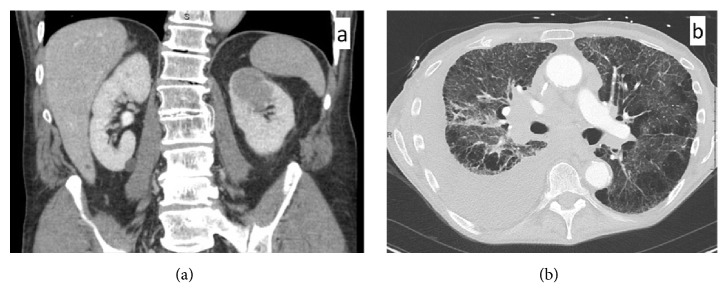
(a) Contrast enhanced CT shows a 4.5 cm left upper pole renal mass. (b) Chest CT shows an ill-defined obstructive right perihilar mass, which is inseparable from the extensive mediastinal and hilar lymphadenopathy. There is irregular and nodular inter-/intra-lobular septal thickening predominantly in the right middle and lower lobe with ipsilateral pleural effusion, suggestive of lymphangitic carcinomatosis.
